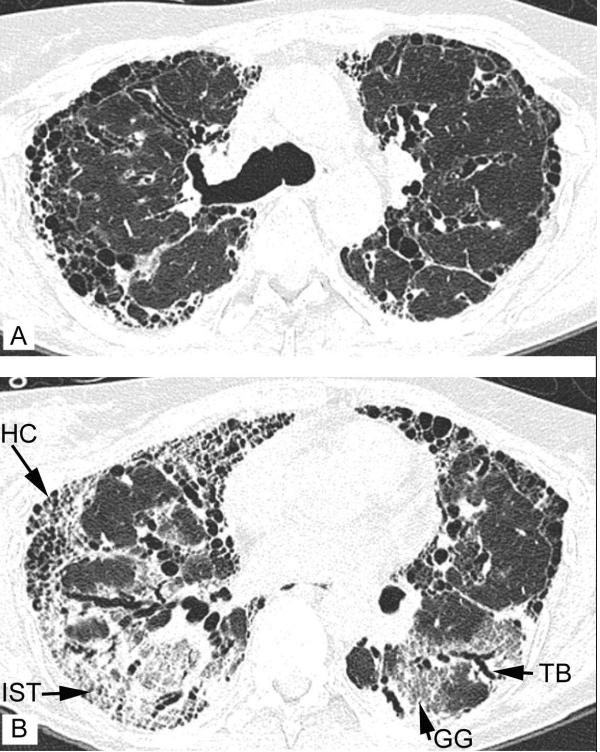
High resolution computed tomography (HR-CT) of the lung showing characteristics of fibrosis. (a) HR-CT at the level of the main carina. (b) HR-CT at the basal parts of the lungs 1 cm above the diaphragm. Inter- and intralobular septal thickening (IST), traction bronchiectasis (TB), honeycombing cysts (HC) and ground glass (GG) are indicated.
